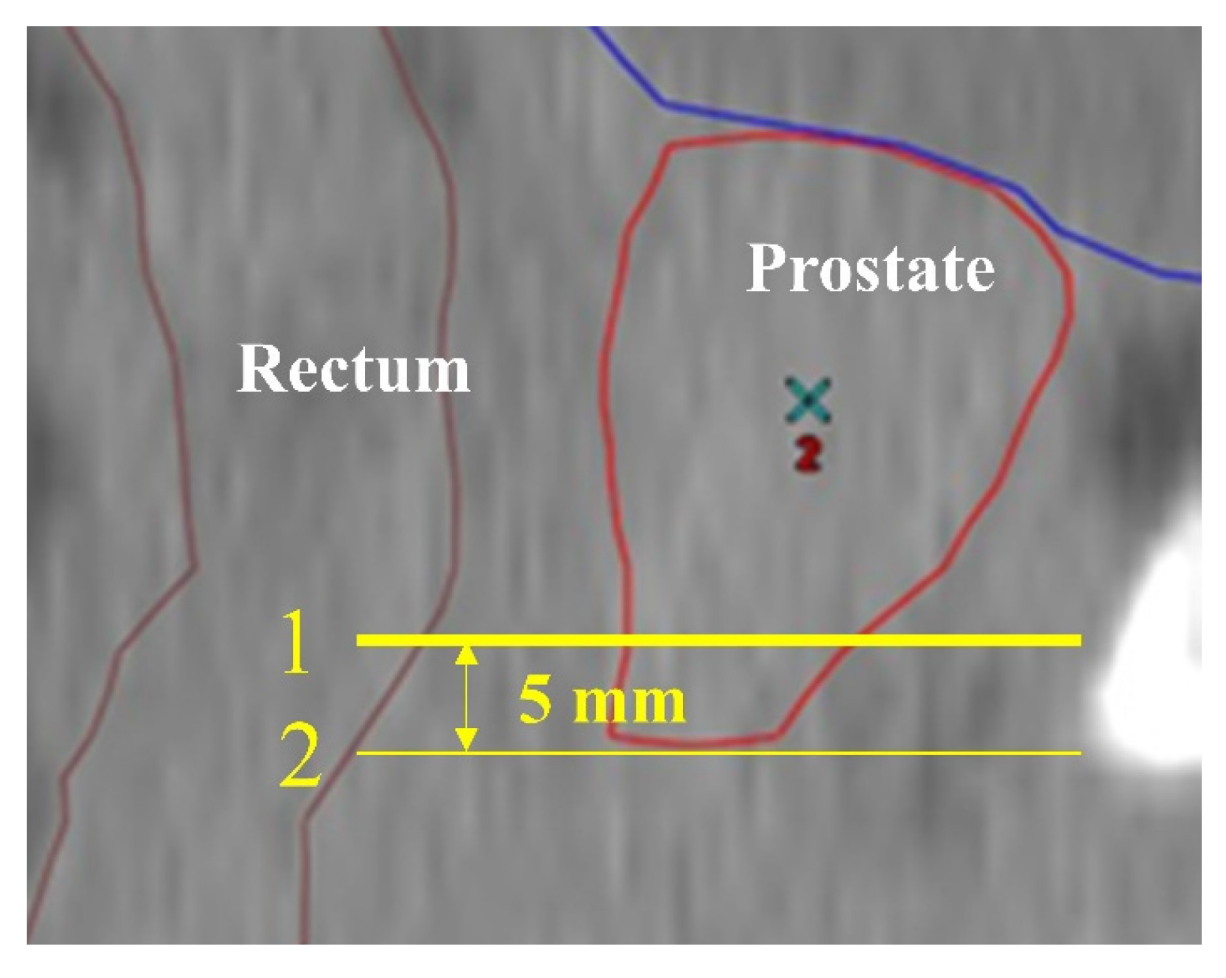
CT scan (sagittal). CT scan taken for radiation therapy planning. Line 2 indicates the level of the edge of the contoured prostatic apex. Line 1 indicates the level 5 mm cranial to Line 2, which is the level of the edge of the prostatic apex. Cases in which the hydrogel spacer could not be identified at the level of Line 1 on the MRI scan taken on the same day as the CT scan for treatment planning were defined as those with the hydrogel spacer not in place at the apex.
